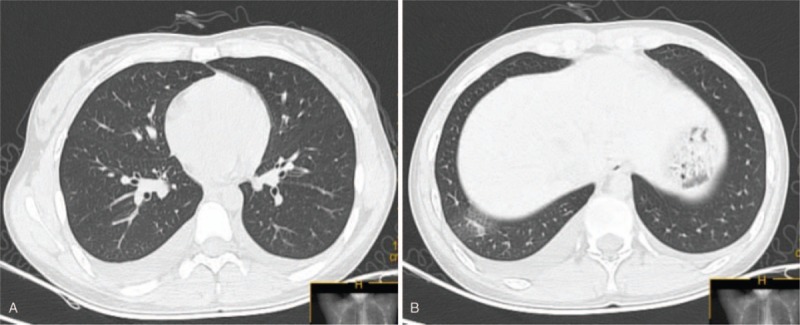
(A, B) Chest computed tomography showed improvement in bilateral infiltrates after 4 days methylprednisolone treatment.
